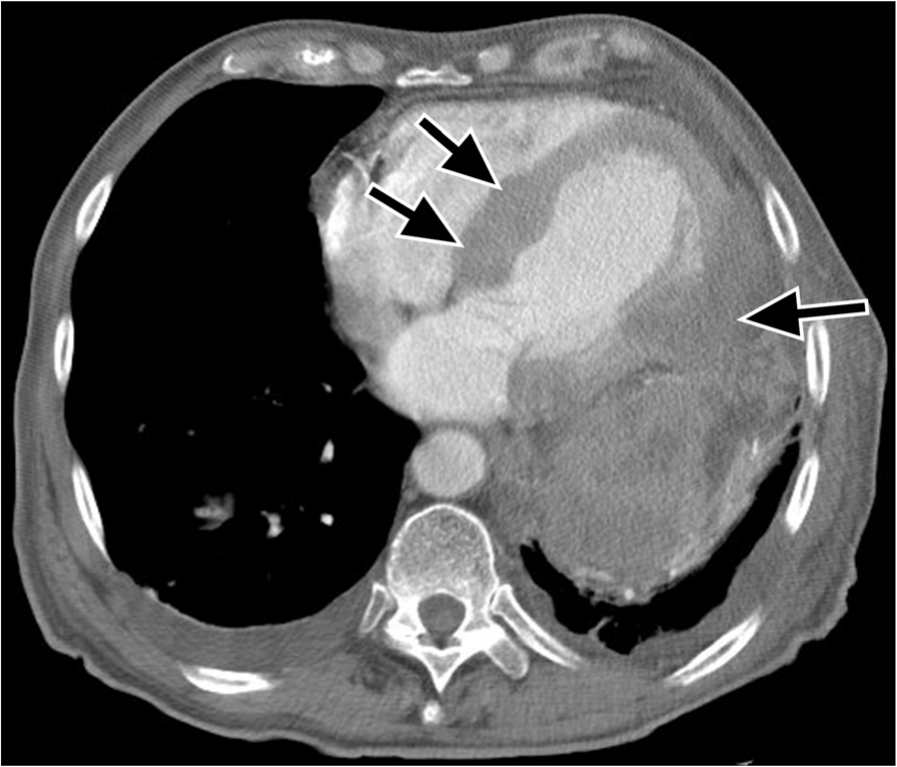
Axial CT with contrast demonstrates nodules and masses (black arrows) in the interventricular septum and the left ventricular free myocardial wall, in a 78-year-old male patient with metastatic malignant melanoma. There is also a large necrotic lymph node in the left mediastinum adjacent to the cardiac implants
